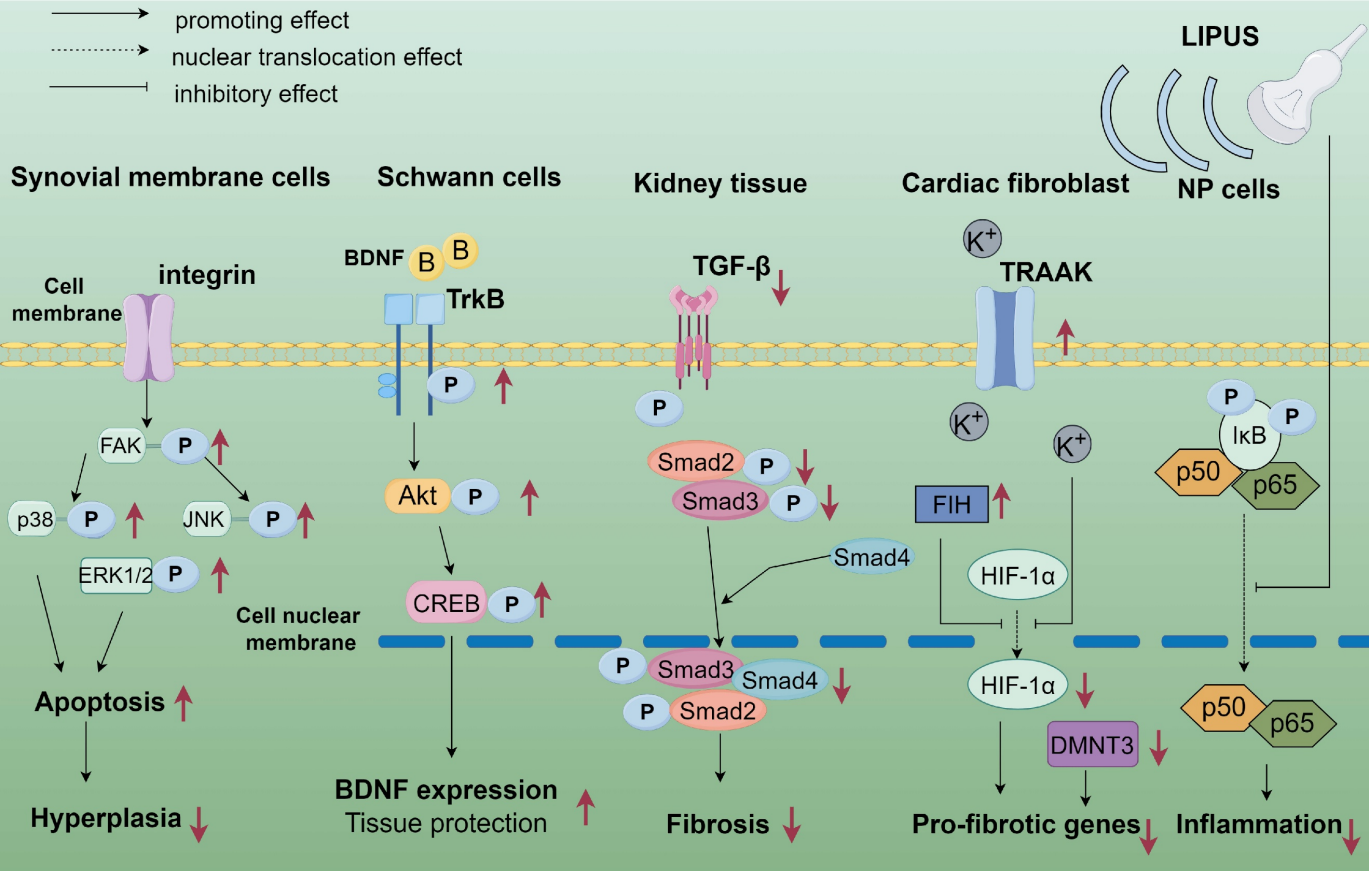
The application of LIPUS in anti-inflammation and repair at the biological and molecular level. LIPUS up-regulated phosphorylated FAK in synovial cells, leading to decreased ERK, c-JNK, and p38 phosphorylation. LIPUS modulated apoptosis and survival of osteoarticular synoviocytes through the integrin/FAK/MAPK signaling pathway. LIPUS activates SCs through the TrkB/Akt/CREB axis in peripheral nerves, enhancing SCs proliferation, migration, and nerve growth factor expression. In an adriamycin-induced chronic renal injury model, LIPUS treatment significantly ameliorated renal injury by blocking signaling between TGF-β1 and its downstream molecules, Smad2 and Smad3 to reduce fibrosis and inflammation. LIPUS partially mitigated hypoxia-induced cardiac fibroblast phenotypic changes through the TRAAK-mediated HIF-1α/DNMT3a signaling pathway. In LPS-induced NP cells, LIPUS inhibited the NF-κB signaling pathway by suppressing p-P65 protein expression and P65 translocation to the nucleus, i.e., inhibiting the NF-κB signaling pathway, inflammation, and catabolism in LPS-induced human degenerative medulla oblongata cells. By Figdraw. Abbreviation: CREB: cyclic-AMP response binding protein; DNMT3a: DNA methyltransferase3α; ERK: extracellular regulated protein kinases; HIF: hypoxia-inducible factor; JNK: c-Jun N-terminal kinase; LIPUS: low intensity pulse ultrasound; MAPK: mitogen-activated protein kinase; NF-κB: nuclear factor-κB; NP: nucleus pulposus; SCs: Schwann cells; TrkB: tyrosine Kinase receptor B; TGF-β: transforming growth factor-β; TRAAK: TWIK-related arachidonic acid-activated K^+^ channel.
